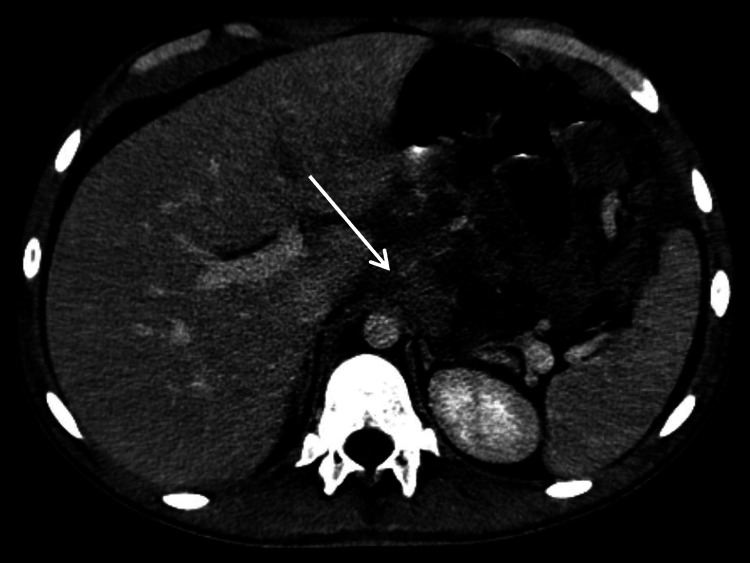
Abdominopelvic CT demonstrating multiple enlarged lumbar-aortic and mesenteric lymph nodes. The arrow indicates confluent adenopathic clusters surrounding the celiac trunk, suggestive of lymphoproliferative involvement. CT: computed tomography
